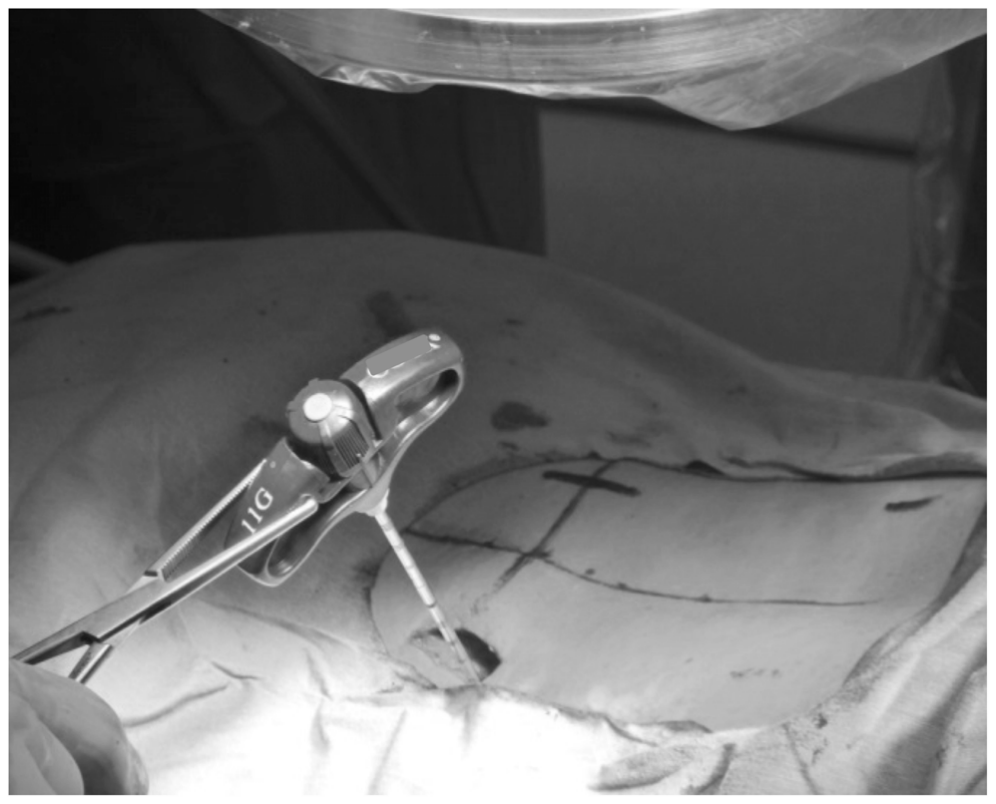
Protective techniques against excessive radiation exposures. Surgeons should always pay attention to the location of their hands at every fluoroscopic shots during MIS-TLIF procedures. The hands are often exposed to the X-ray beam while confirming the insertion point of the Jamshidi needle. A long Kocher clamp can be used to hold the Jamshidi needle for keeping a distance from the X-ray tube.
